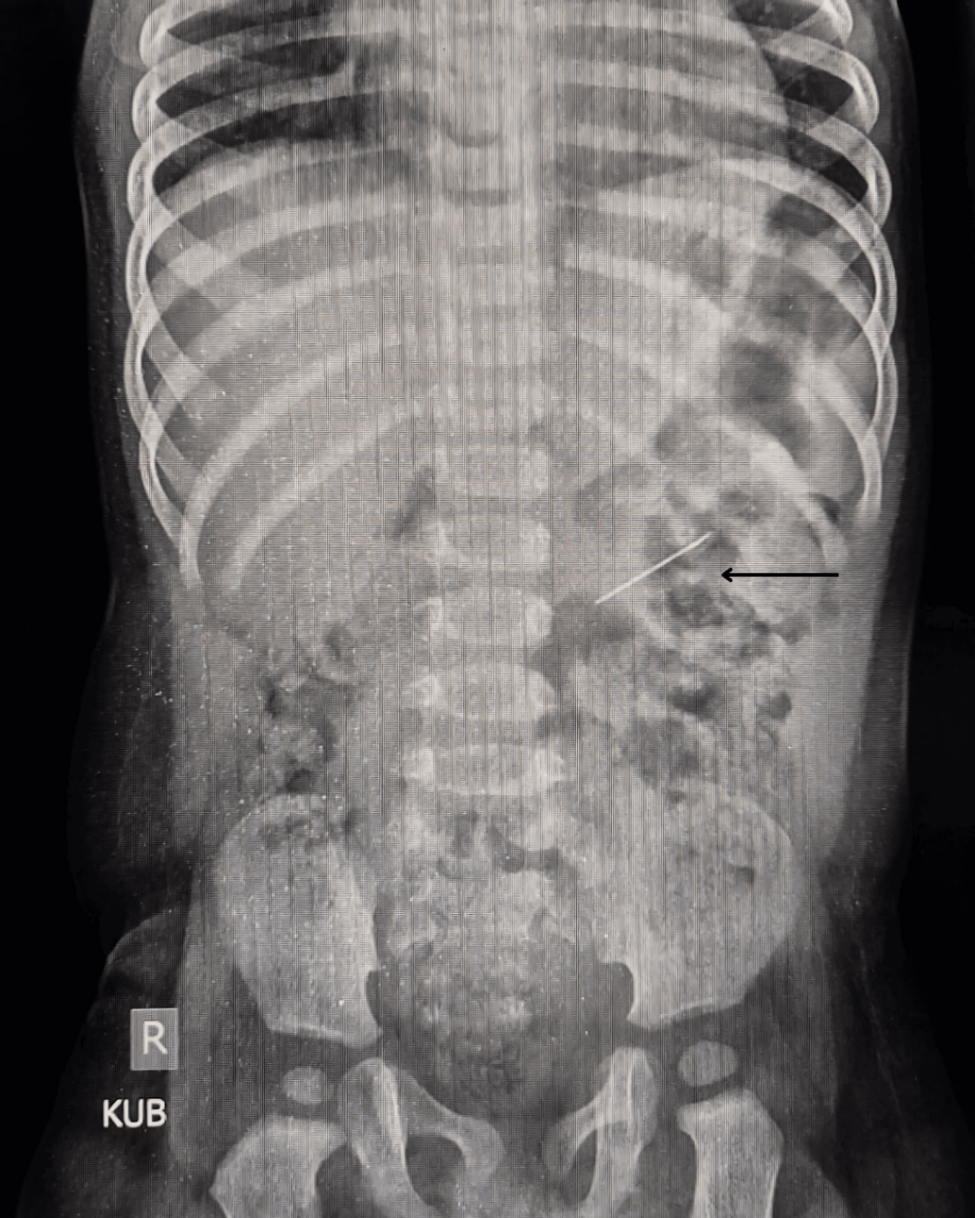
X-ray KUB showing a linear radio opaque foreign body in the left lumbar region. KUB: Kidney and urinary bladder
